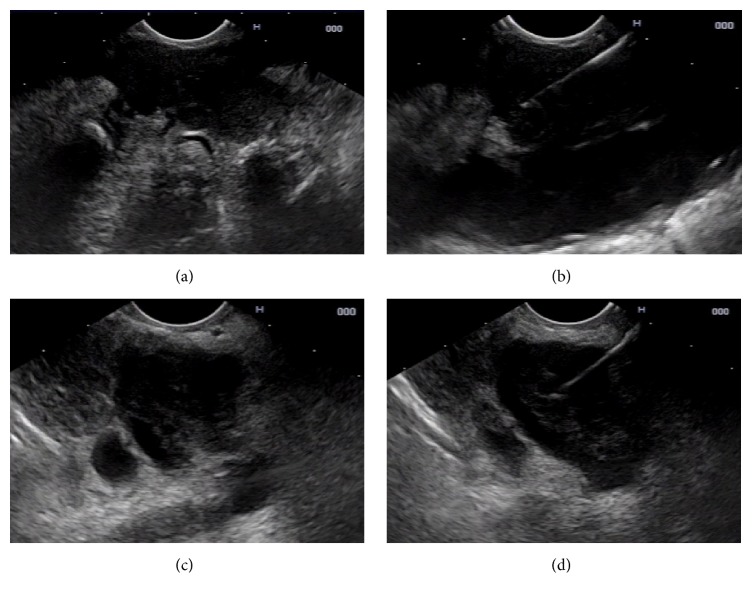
(a) Endoscopic ultrasound (EUS) demonstrating a 3 cm hypoechoic mass in the left lung. (b) EUS-guided fine needle biopsy (FNB) of the left lung nodule with a 25-gauge needle. (c) EUS demonstrating a 3.8 cm hypoechoic mass in the pancreatic body abutting the splenic artery. It shows the same internal echotexture as the lesion in the mediastinum. (d) EUS-FNB of the pancreatic mass with a 25-gauge needle.
